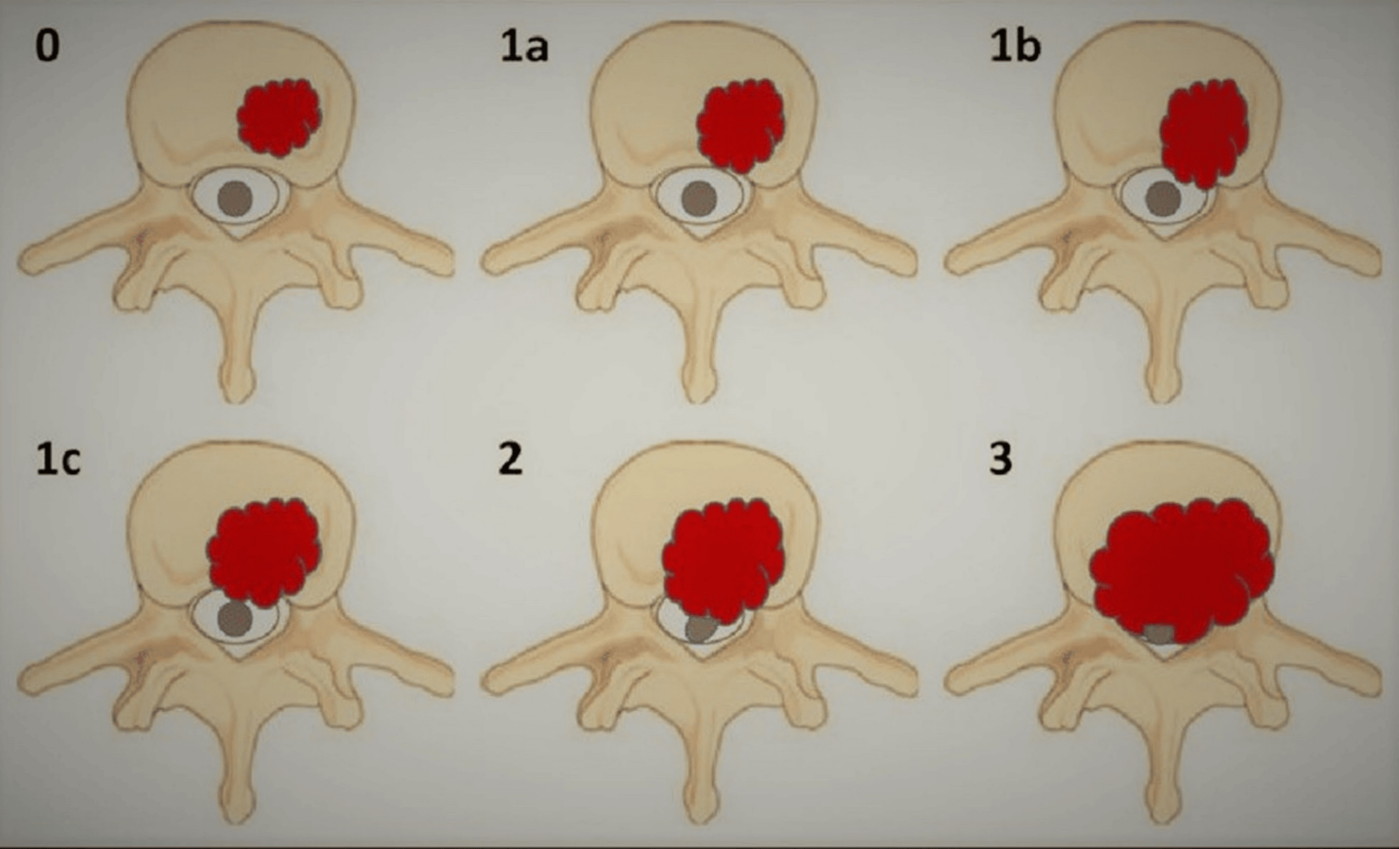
Grading of Epidural Spinal Cord Compression Using the Bilsky Criteria T2-weighted MRI images showing grades 0-3 of spinal cord compression. Grade 0: No deformation; Grade 1: Epidural impingement without spinal cord compression; Grade 2: Spinal cord compression with visible cerebrospinal fluid (CSF); Grade 3: Spinal cord compression with no visible CSF. Figure credit: Reproduced with permission from Zaveri et al. [18].
